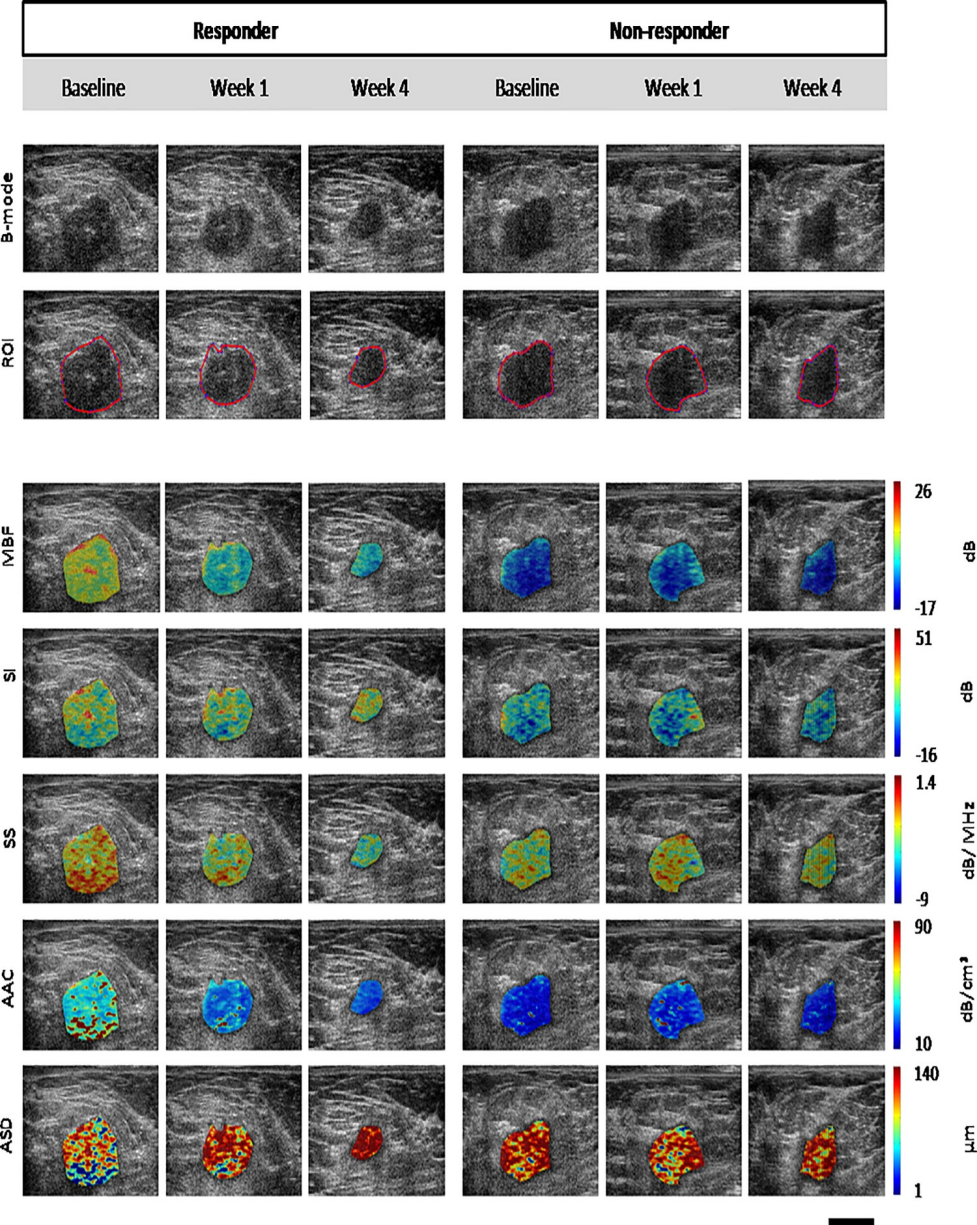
Ultrasound B-mode and QUS-derived parametric maps for representative responder and non-responder patients (responder—left, non-responders—right panel) acquired at baseline, and weeks 1 and 4 of treatment. Abbreviations: MBF (dB): mid-band fit, SS (dB/MHz): spectral slope, ASD (μm): average scatterer diameter, AAC (dB/cm^3^): average acoustic concentration, SI (dB): spectral intercept. Scale bar represents 2 cm.
